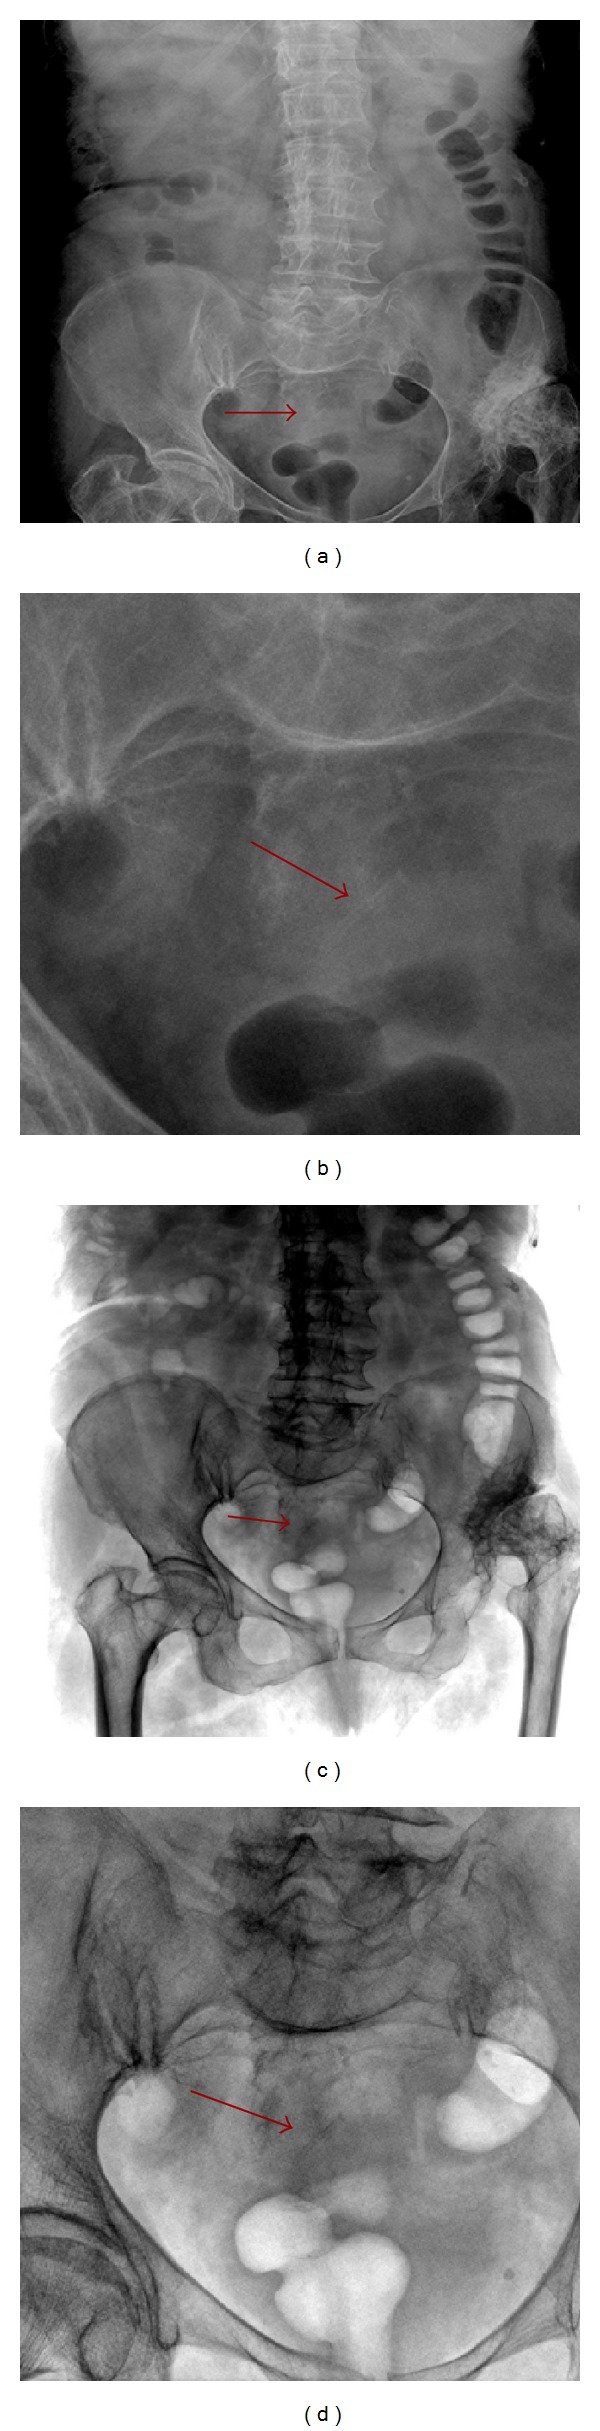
Plain digital radiographs made in January previous hospitalization in another institution and evaluated again in May after window stressing (a, b) and with inversion effect (c, d) showing that the foreign body was already present but not easy to be demonstrated without clinical suspicion due to its low radiopacity.
